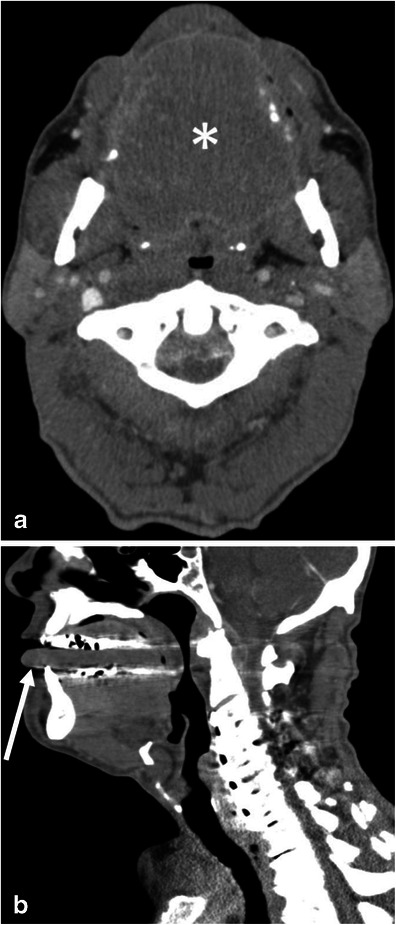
A 64-year-old male with amyloidosis presenting with diffuse tongue swelling. a The axial CE-CT shows the tongue to be diffusely swollen (*asterisk*). Note the attenuation of the tongue is soft tissue density. Glossomegaly caused by angioedema typically shows low density (see Figs. 6 and 7). b The sagittal CE-CT shows diffuse tongue swelling with anterior protrusion of the tip of the tongue (*arrow*)
